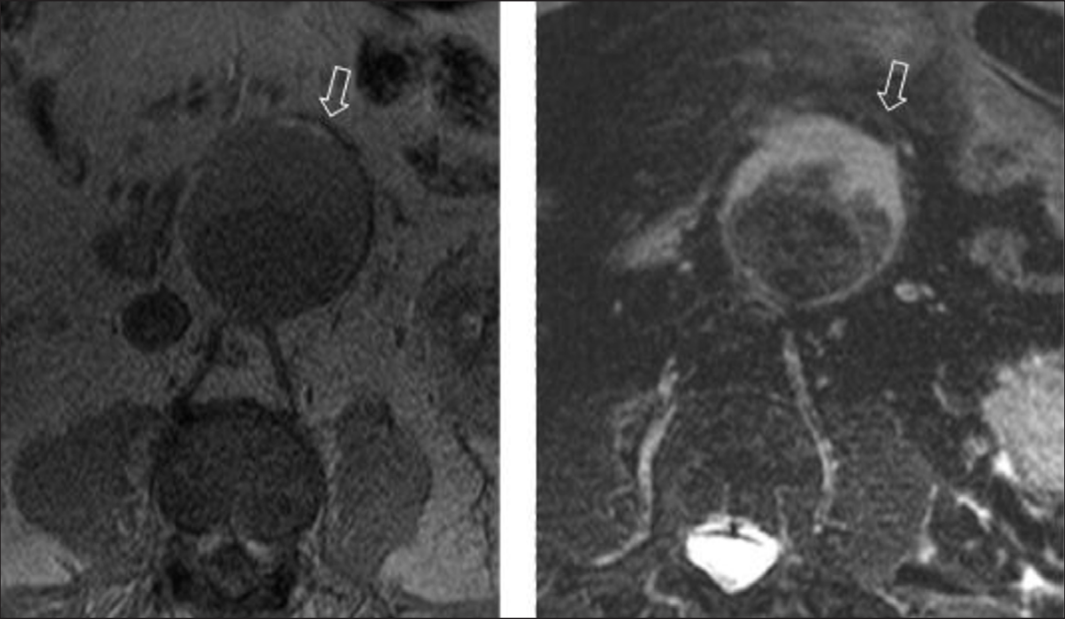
Transverse MRI of a large but stable AAA using a T1-weighted (left panel) and a T2-weighted image with fat saturation (right panel) in which the fat appears with high and very low signal, respectively (open arrows).
